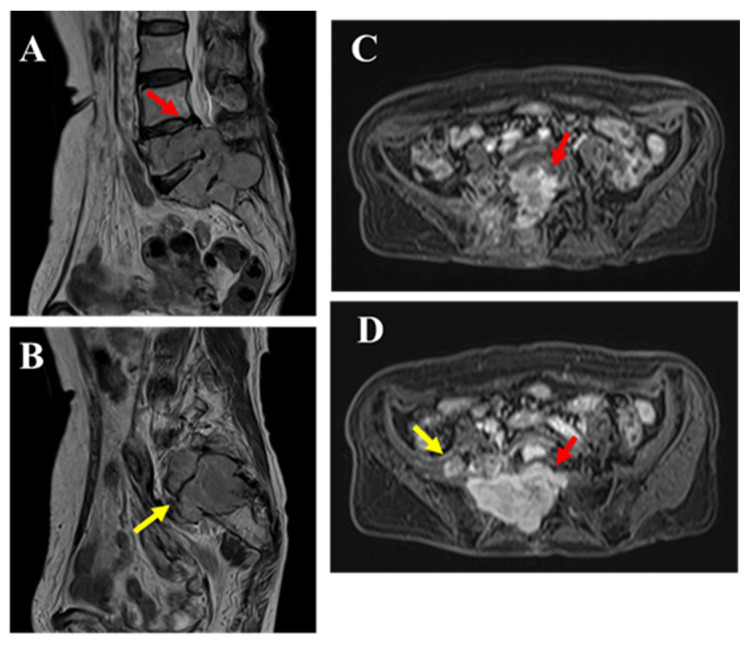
MRI revealed that although the mass had increased in size over time, its T2 signal intensity (A,B the mass within the spinal canal (red arrow) and outside the intervertebral foramen (yellow arrow)) and contrast enhancement (C,D the mass within the spinal canal (red arrow) and outside the intervertebral foramen (yellow arrow)) showed no significant changes compared to the initial imaging. Bone invasion was evident, and pathological fractures were observed in the affected bone. An open biopsy was performed to confirm the diagnosis.
